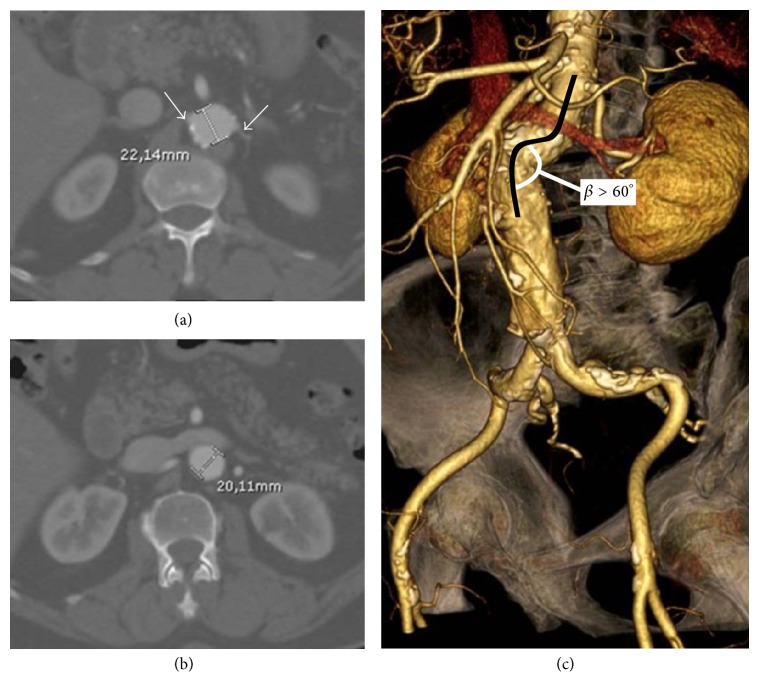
Preliminary CT-A showing (a, b) the reversed tapered shape of the proximal neck (arrows: renal arteries origin) and the severe *β*-angle (c).
